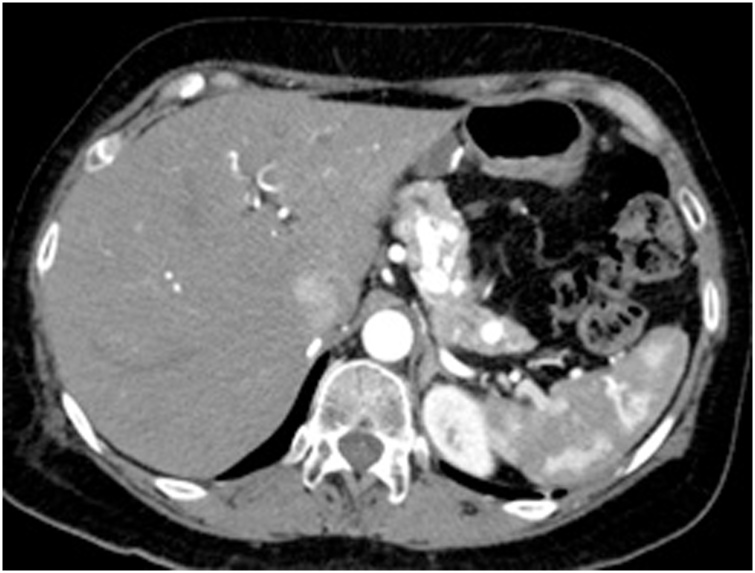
CT scan showing multiple metastatic lesions in the pancreas post Whipple procedure.
